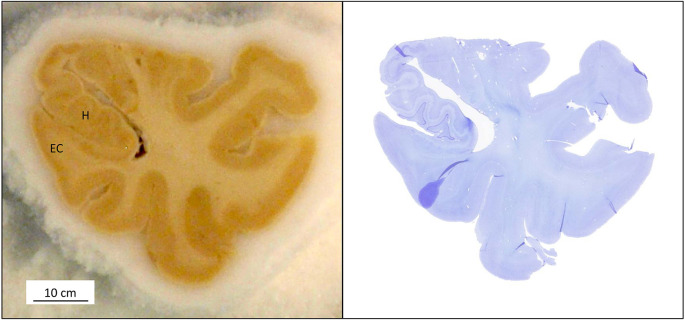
(Left) A sectioned block of the temporal lobe is positioned on the microtome stage with the medial side of the hippocampus (H); EC: entorhinal cortex. (Right) The Nissl and MRI correspondence are shown at the same level.
